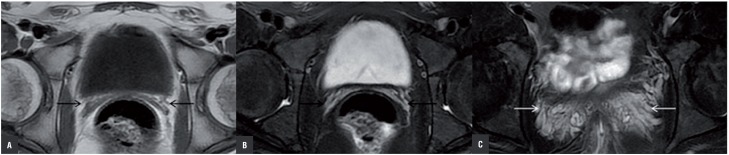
Bilateral SV hypoplasia in an 18 year old male patient with complaints of pelvic pain. Axial (a) T1 weighted MR image and axial (b, c) fat-saturated T2 weighted MR images show both SV were significantly small (black arrow). Congestion were visualized in pelvic vascular structures at the SV location (white arrow).
